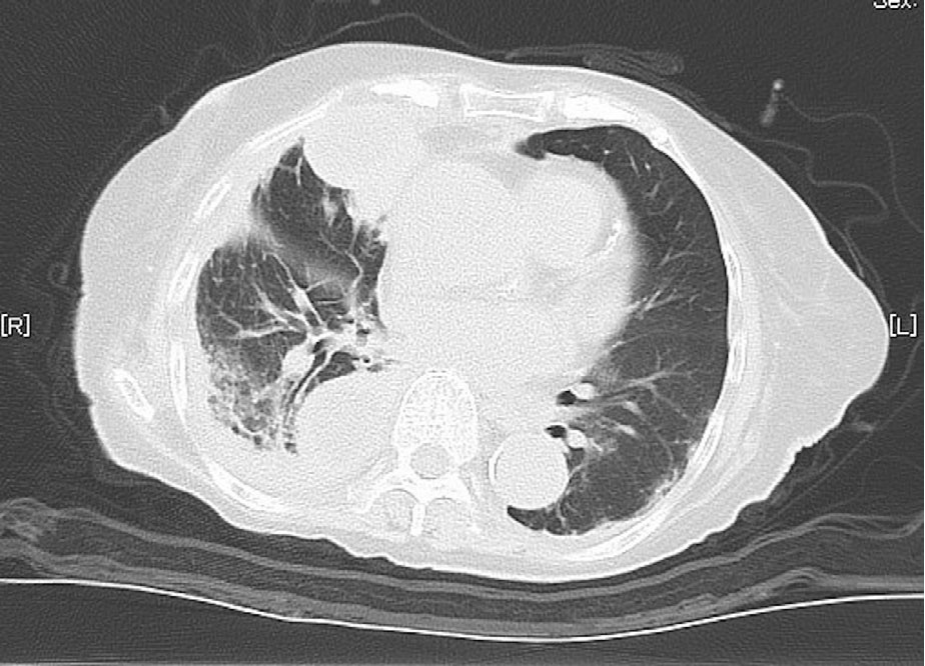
Chest CT scan images upon admission reveal the presence of a pyothorax cavity located in the lower lobe of the right lung, extending from near the spine to the base of the right lower lobe.
